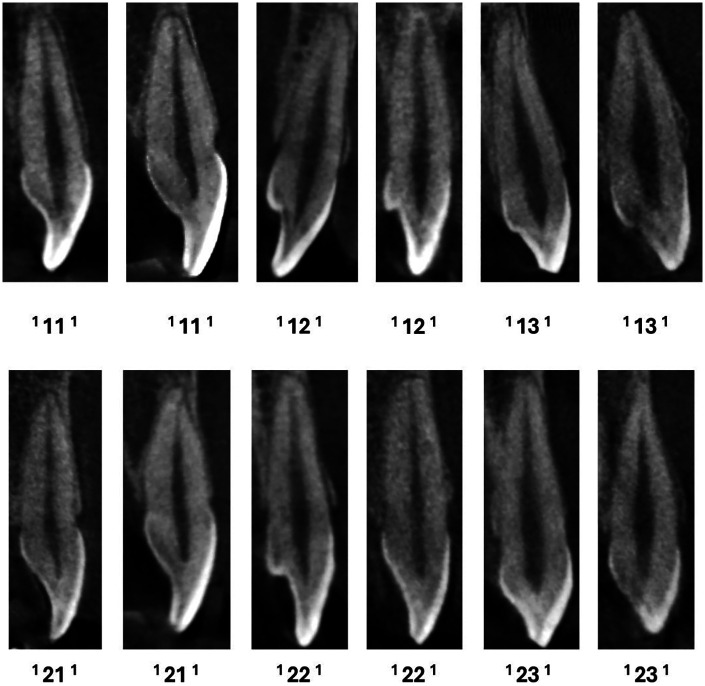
CBCT sagittal view of maxillary anteriors with a single canal.
